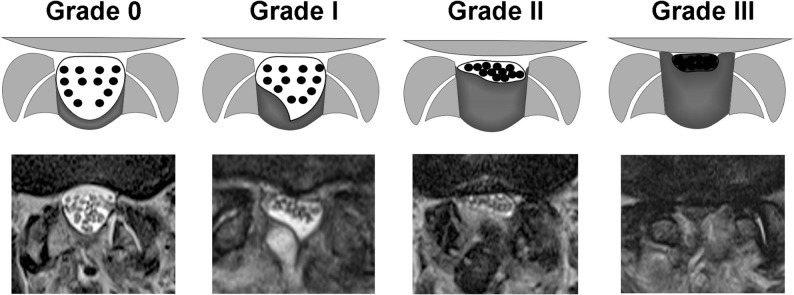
Classification of postoperative spinal epidural hematoma on axial T2-weighted MRI. Grade 0 – No hematoma. The dural sac appears round with clearly visible CSF. Grade I – Mild dural sac compression. Nerve roots are separated and identifiable, with preserved CSF signal. Grade II – Moderate compression. Nerve roots are crowded but still individually recognizable, with partial CSF preservation. Grade III – Severe compression. CSF is absent and nerve roots appear indistinct or obscured
